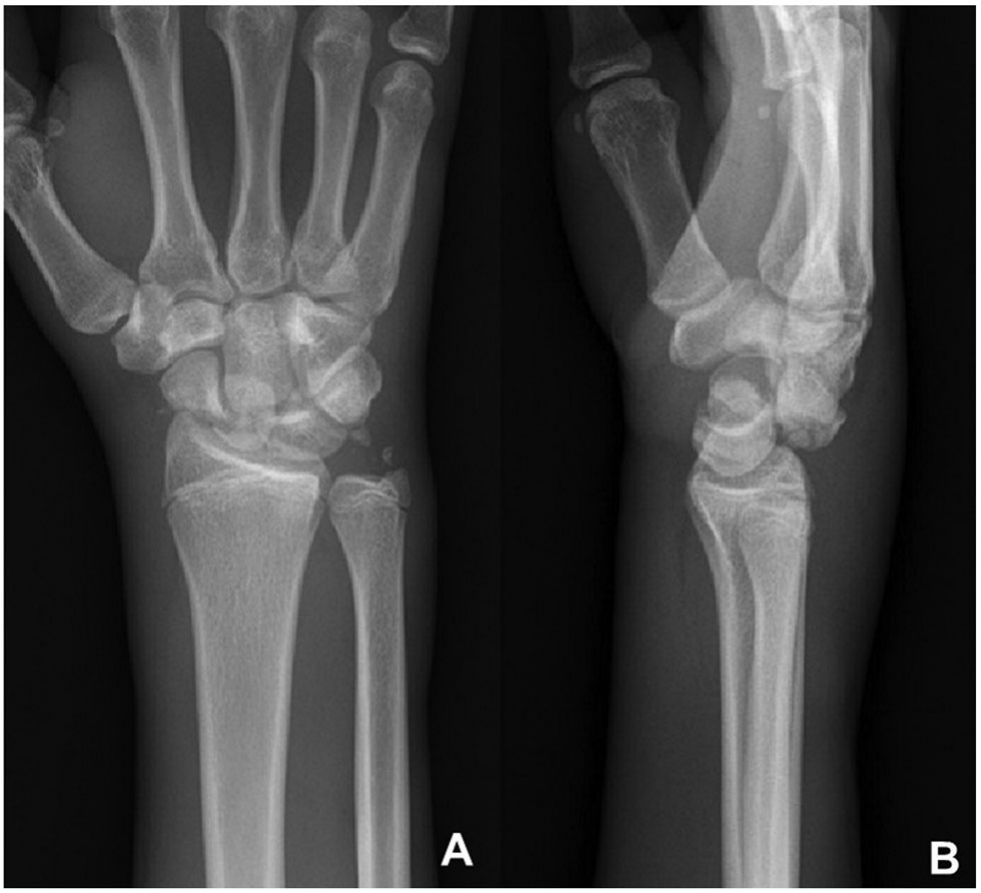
(A and B) Pre-operative x-rays of the first case.
